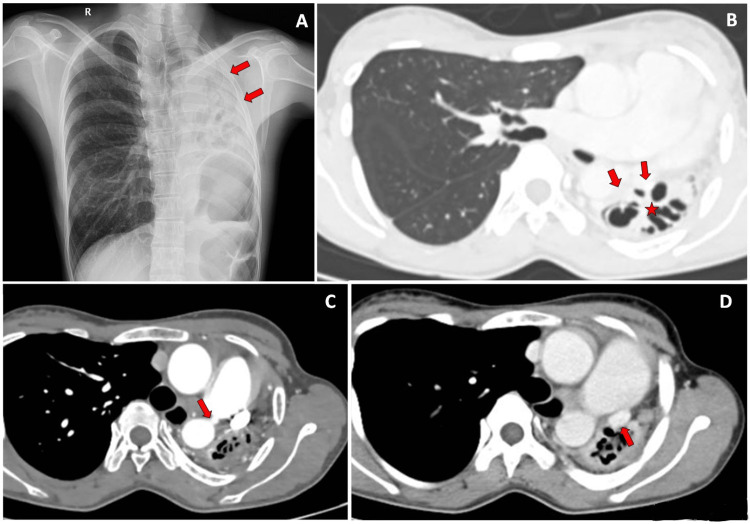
Proximal arrest of left pulmonary artery with patent ductus arteriosus anomaly. A 20-year-old female presented with complaints of recurrent upper respiratory tract infections. On Chest Radiography, hypoplastic left upper lobe was noted. On CECT Chest examination, hypoplastic left upper lobe with cystic bronchiectasis was noted. The ductus arteriosus was noted to be patent along with a persistent left superior vena cava. 5A: Chest X-Ray shows hypoplastic left lung (red arrows). 5B: Chest CECT of the patient demonstrates hypoplastic left lung (red arrow) with cystic bronchiectasis (red star). 5C: Chest CECT of the patient demonstrates patent ductus arteriosus (red arrow). 5D: Chest CECT of the patient demonstrates persistent left superior vena cava (red arrow). CECT: Contrast Enhanced Computed Tomography
